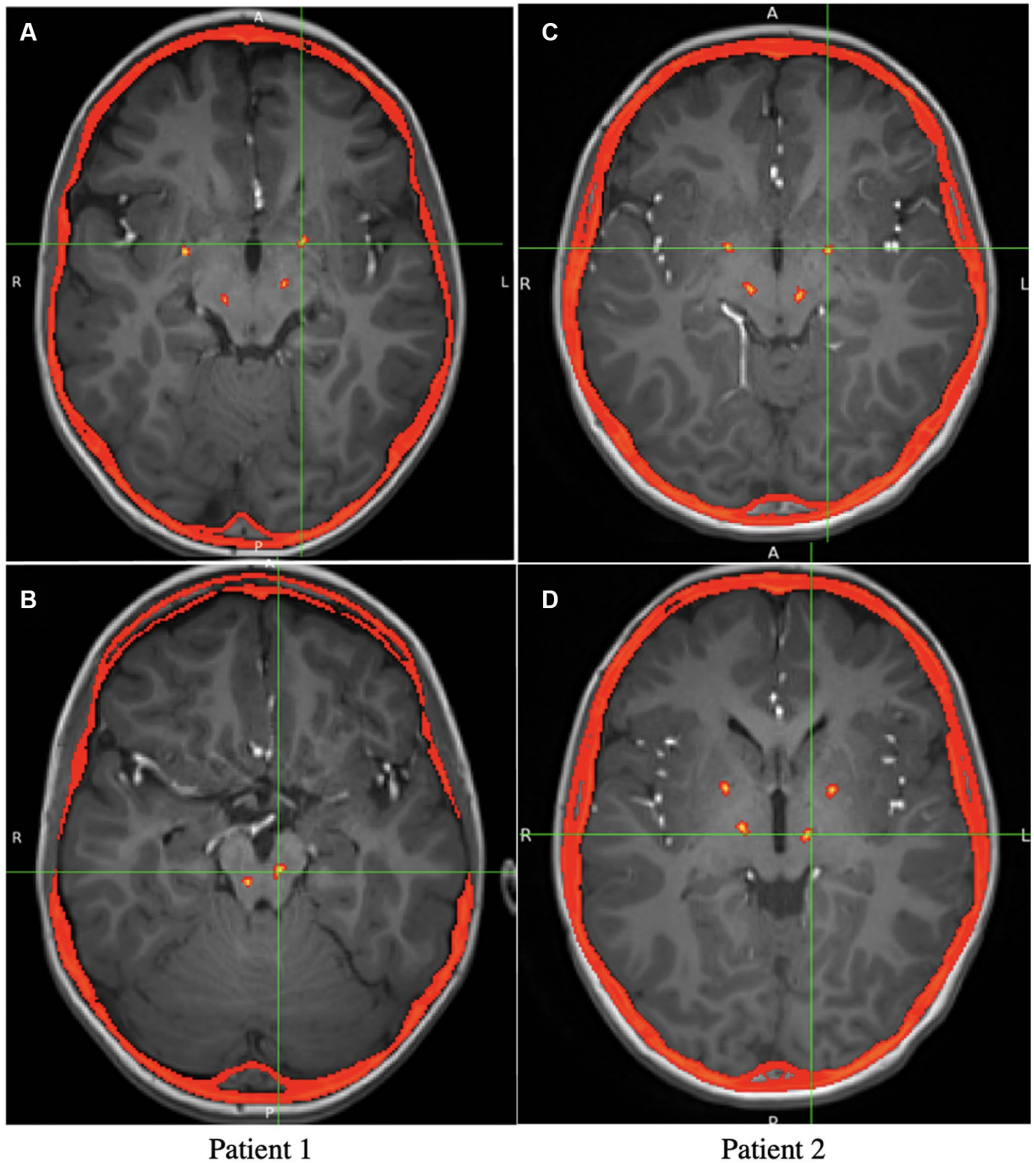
Axial view of the postoperative CT overlaid on preoperative MRI, showing the locations of the Medtronic leads. (A) Axial view of the GPi electrodes in patient 1 (The PPN electrodes are also visible but not at the target). (B) Axial view of the PPN electrodes in patient 1. (C) Axial view of the GPi electrodes in patient 2 (The VIM electrodes are also visible). (D) Axial view of the VIM electrodes in patient 2 (The GPi electrodes are also visible).
